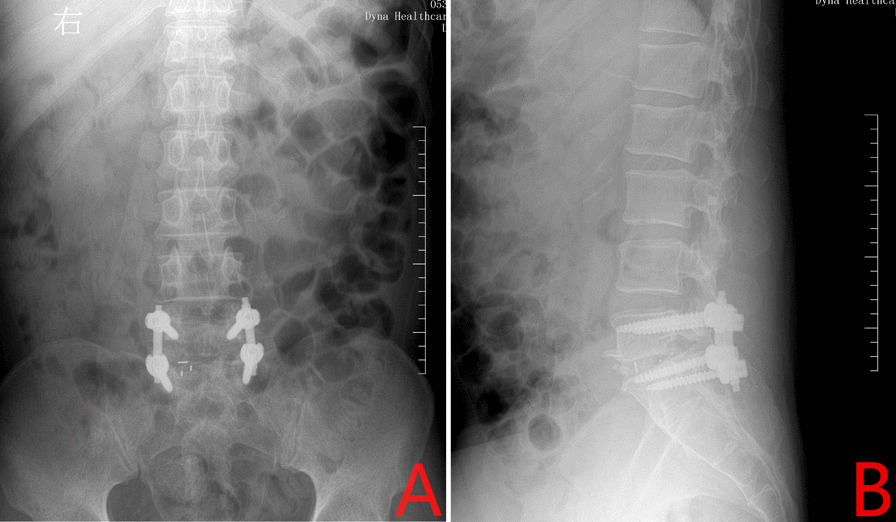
Postoperative radiography. A Post-operative anteroposterior X-ray image. B Postoperative lateral x-ray image taken on the 3rd postoperative day. The fusion cage and internal fixation device between the 5th lumbar and 1st sacral vertebral bodies can be seen
